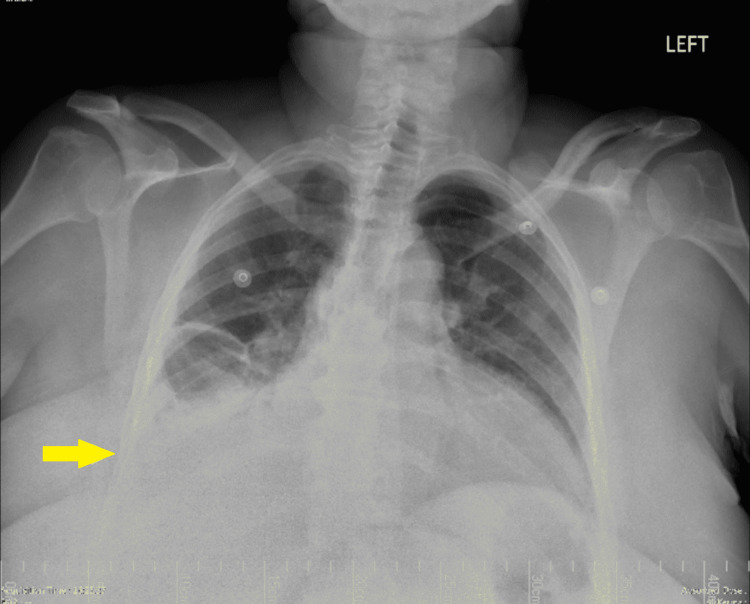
Chest x-ray showing a right basilar consolidation and effusion (yellow arrow).
